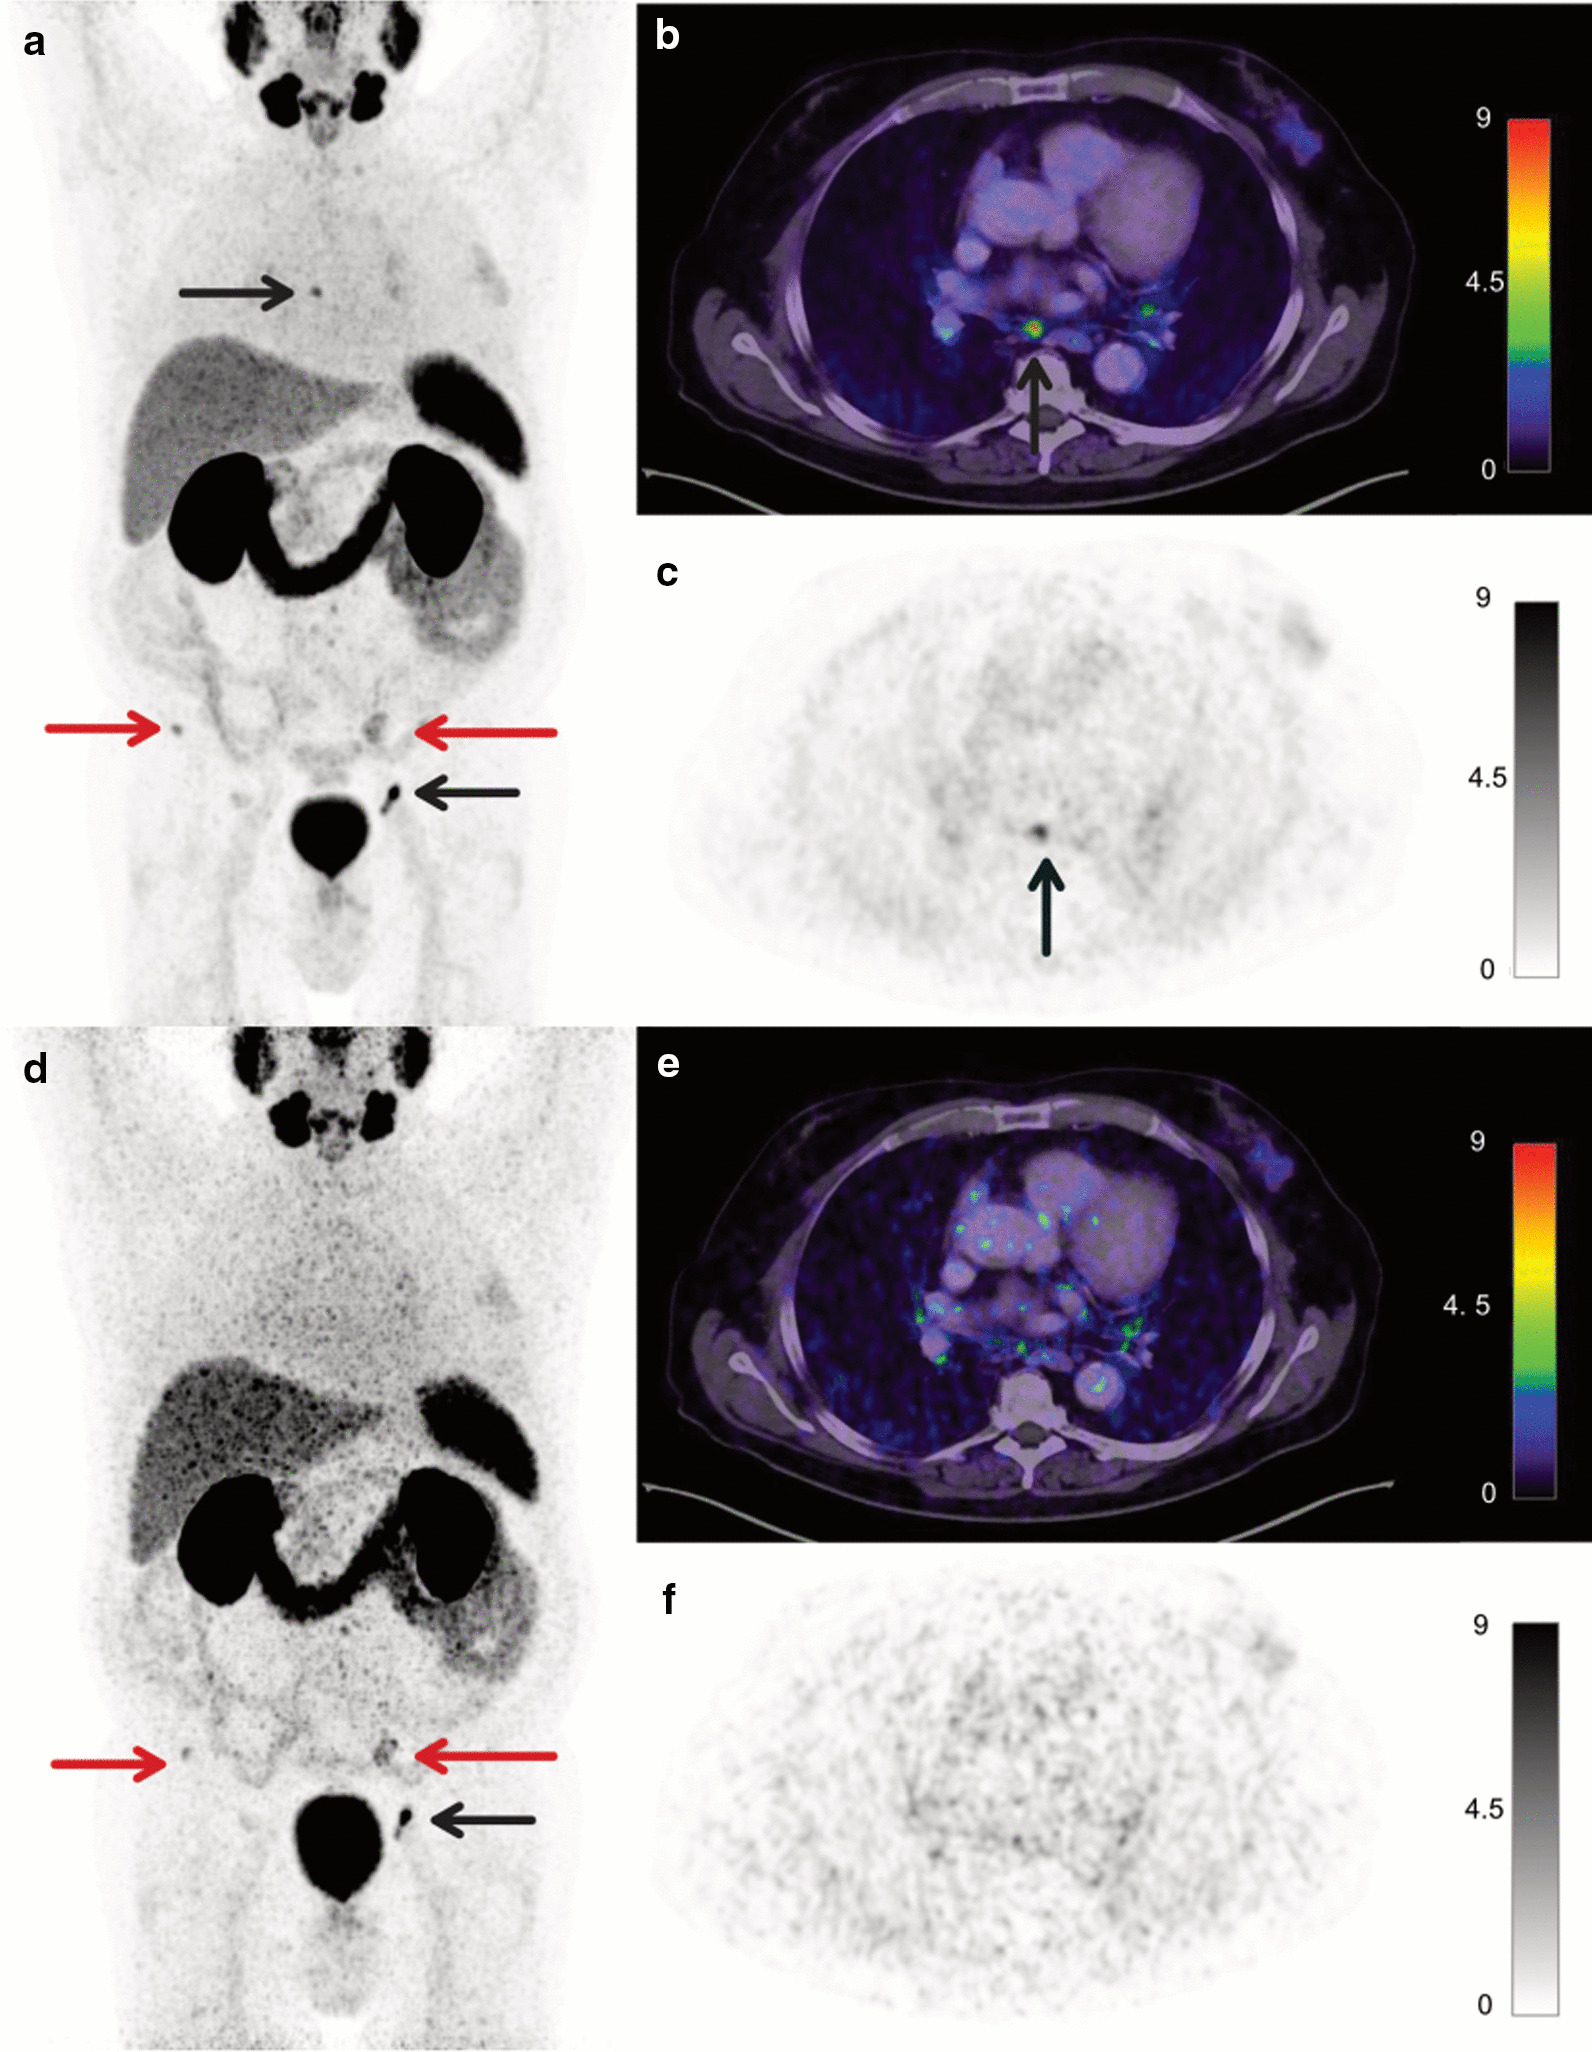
A 65-years old patient (Pat. ID #7) with second biochemical recurrence after primary prostatectomy and salvage external beam radiation therapy. PSA was 0.6 ng/ml at the time of imaging. a–c show images acquired with the reference acquisition protocol; d–f show images acquired with the reduced acquisition protocol, all reconstructed with OSEM-PSF + TOF(4i). Pathological tracer uptake in a mediastinal lymph node visible on the images acquired with reference protocol (c, black arrow) was not reproducible with three and a half-fold reduction in scan time duration (f). Maximum intensity projection (a, d) and axial [^68^Ga]Ga-PSMA-11 PET/CT slices (b, c, e, f) show pelvic and extrapelvic lymph node metastases (black arrows) and bone metastases (red arrows)
